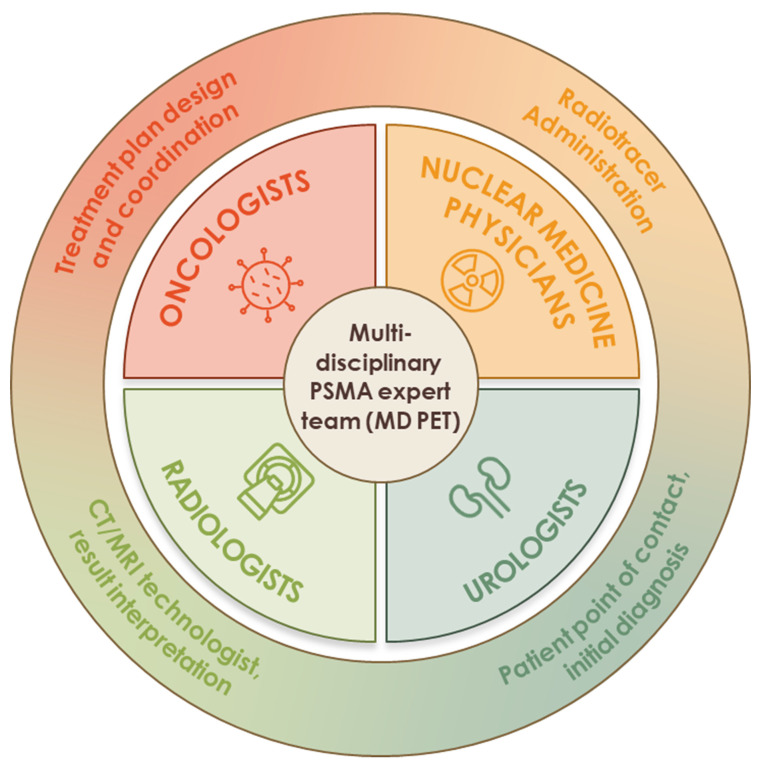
The multi-disciplinary PSMA expert team (MD PET). The multi-disciplinary PSMA expert team (MD PET) consists of 4 core disciplines: oncology, urology, nuclear medicine, and radiology. Multidisciplinary care may improve patient-specific decision-making.
